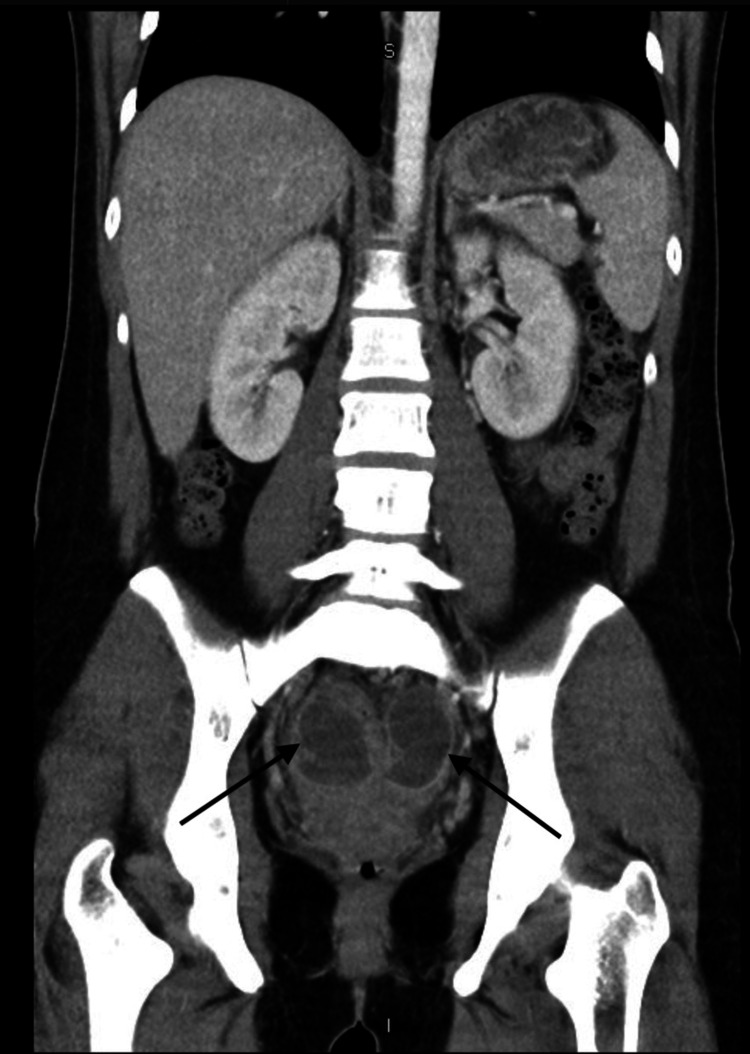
CT abdomen/pelvis (coronal plane) The image is demonstrating bilateral fluid collections (arrows) concerning tubo-ovarian abscesses.
